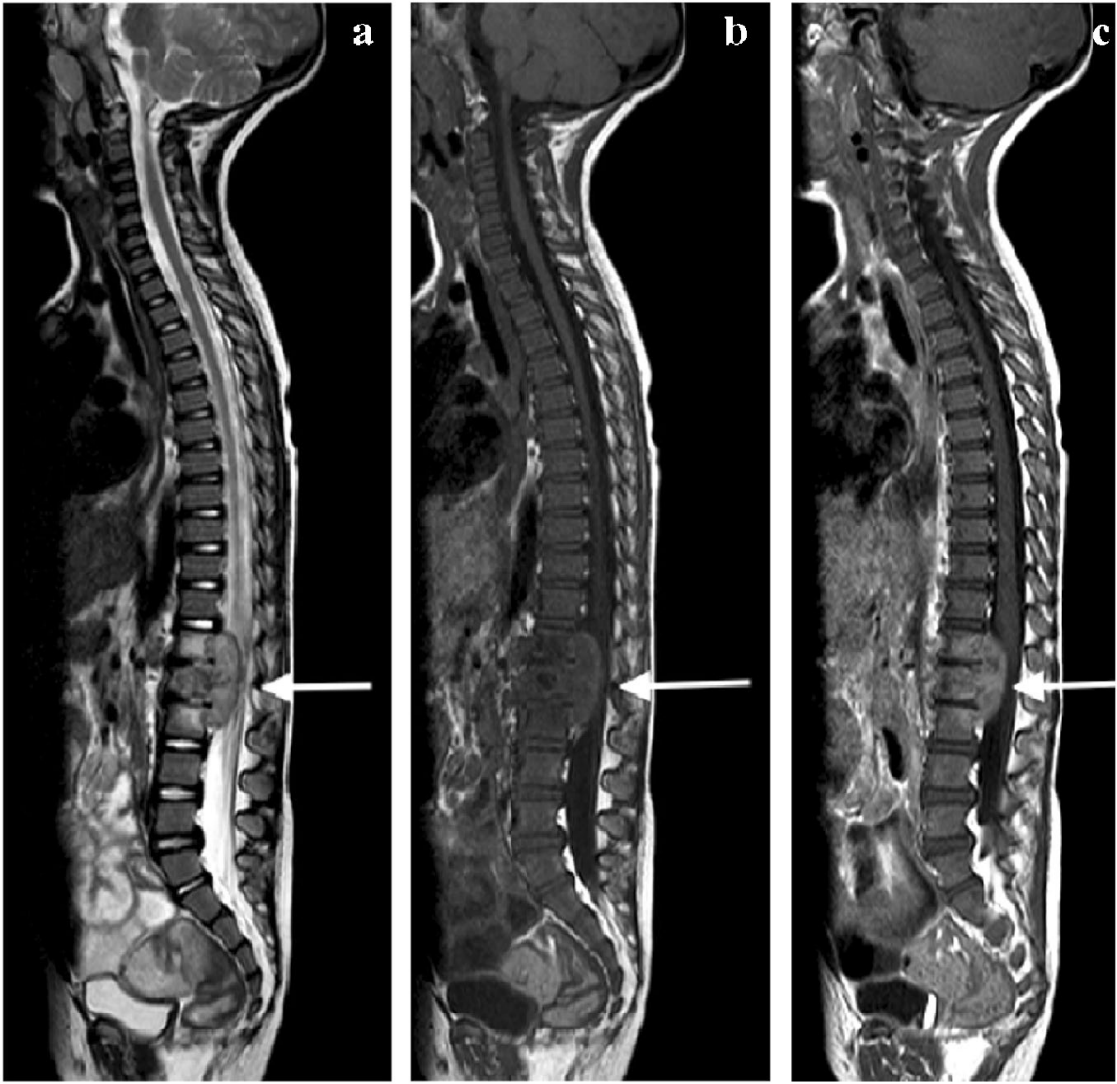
A 2-year-old boy with back pain. a–c Sagittal T2 (a), T1 (b) and T1 post-gadolinium (c) magnetic resonance images of the spine show the involved T12, L1 and L2 vertebral bodies with a soft tissue mass that enhances homogeneously and protrudes posteriorly into the spinal canal compressing the cauda equina (*arrows*). Note the enhancing vertebral bodies and the T2 hypointense non-enhancing discs, also the involvement of the dens of C2 that was not suspected clinically. Tuberculosis was confirmed
